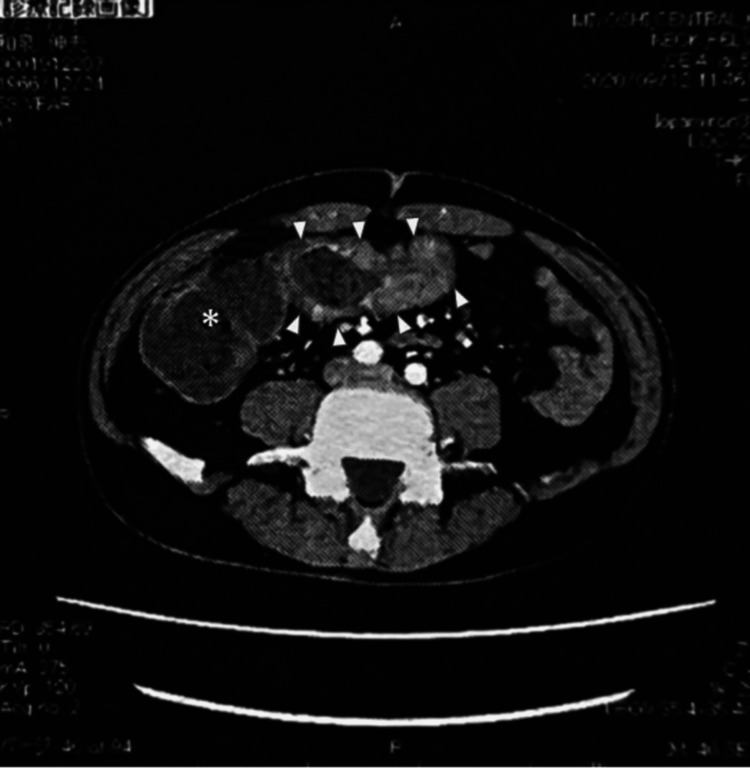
Abdominal CT scan. A circumferentially stenosing tumor 7 cm in length is observed in the transverse colon (arrows). The oral colonic lumen is dilated (asterisk)
